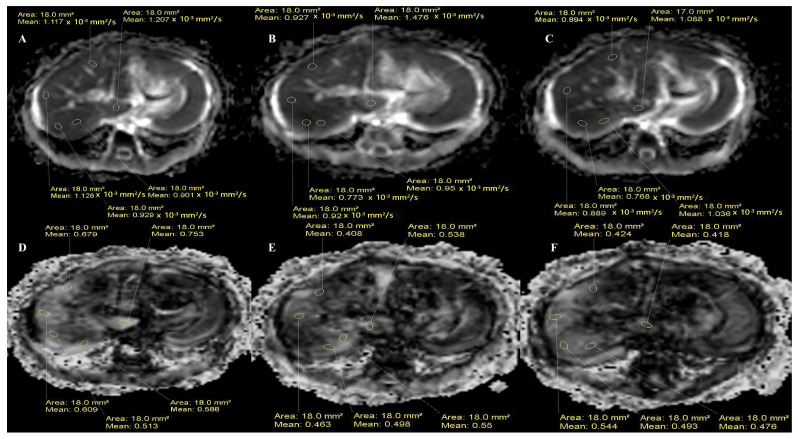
Measurement of fractional anisotropy (FA) and apparent diffusion coefficient (ADC) on diffusion tensor images in a 124-day-old female with biliary atresia using 3T MRI. Three regions of interest (ROIs) were placed on the right hepatic lobe, one ROI was placed on the left and caudate lobes each, and this process was repeated on three consecutive slices at the porta hepatis level. The mean ADC and FA values for the right lobe were calculated from nine ROIs, whereas the left and caudate lobes used three ROIs each. Measurements were performed on ADC (A–C) and FA (D–F) maps for quantitative analysis.
